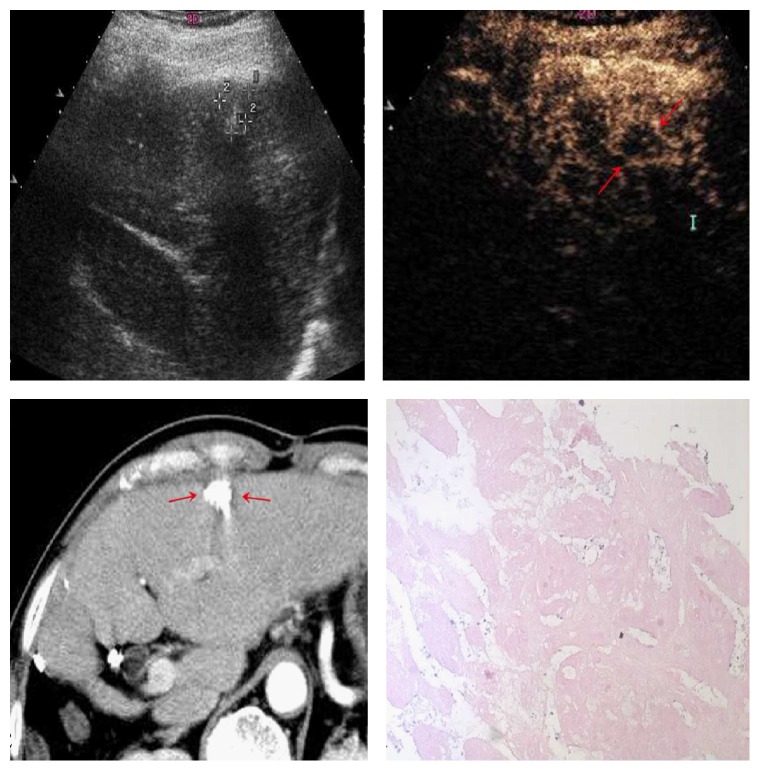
35-year-old man with 1.6 cm×1.3 cm lesion (cross mark). CEUS images in the arterial phase (a, b) showed peripheral rim-like arterial phase enhancement (red arrows), probably representing reactive hyperemia, while the CT examination showed complete lack of enhancement (c) (red arrows). Pathological results showed complete necrosis of the tumor (d).
